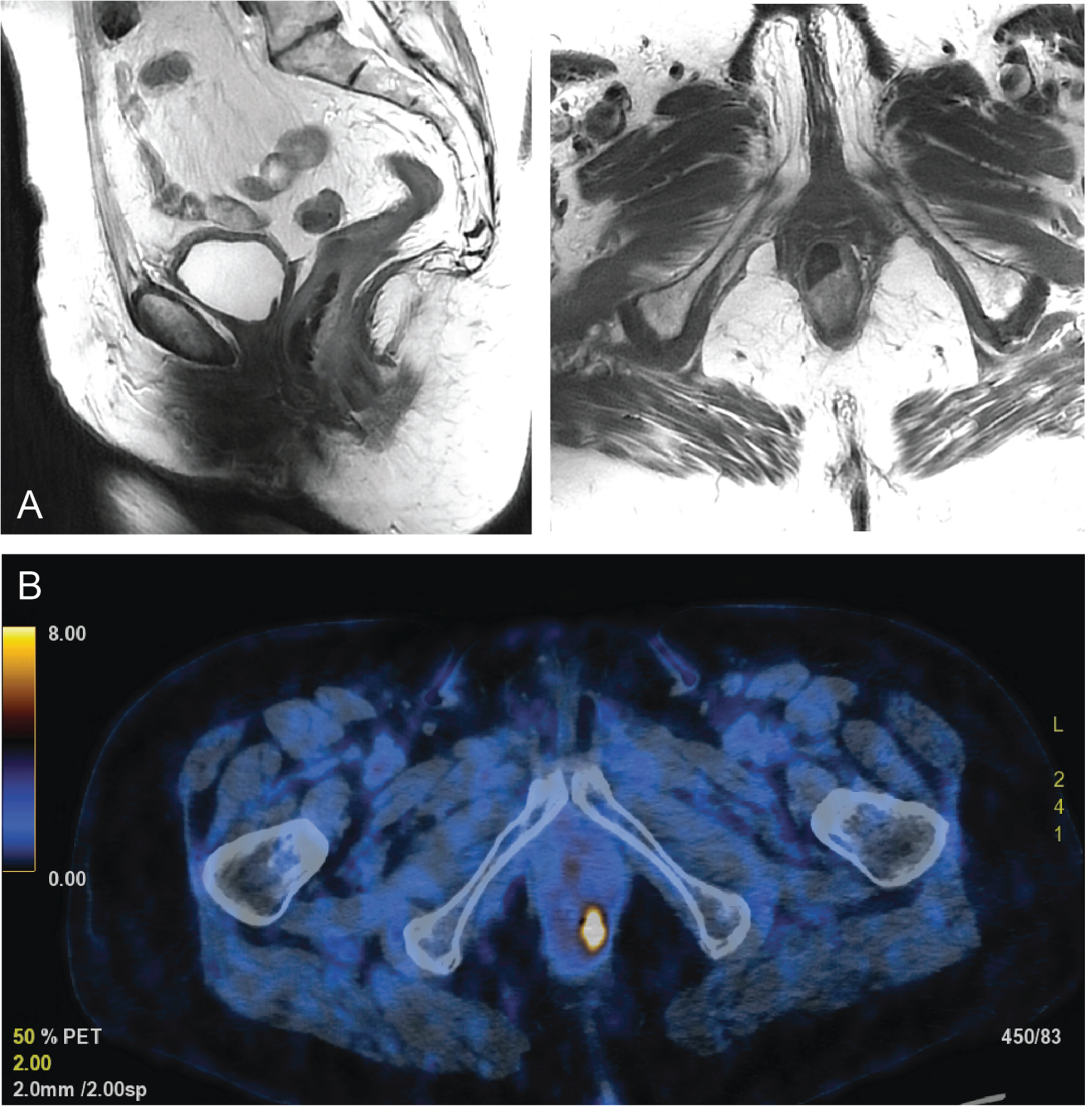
a MRI pelvis: 4 cm long thickening of the dorsal distal rectum wall without signs of sphincter infiltration; b 18F-FDG PET-CT: Metabolic active lesion at the anorectal transition without signs of lymphatic or distant metastasis
